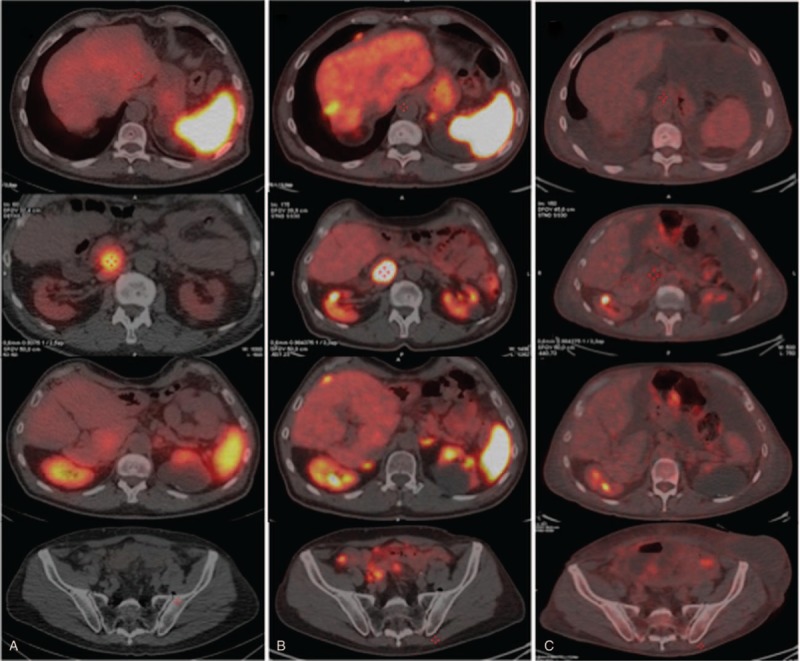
Restaging with ^111^In-Octreotide SPECT/CT scan (column a), ^68^Gallium-DOTATOC (column b), and ^18^F-FDG PET/CT scan (column c) of the patient, performed after colectomy and partial right hepatectomy and subsequent treatment by “cold” somatostatin. Since NET is a G2 tumor (Ki67 index 12%), positivity can observed in columns a and b. ^111^In-Octreotide SPECT-CT scan (column a) showed persistence of the retropancreatic uptake without other significant uptake areas. ^68^Ga-DOTATOC PET/CT scan (column b) confirmed uptake behind the pancreas head (SUV max 56.8) and showed multiple nodules of peritoneal implants in the periglissonian space, omentum, mesenteric region and pelvis (SUV max 13.8-15.4). ^18^F-FDG PET/CT scan (column c): metabolic activity in retropancreatic lesion was absent (SUV max 1.9) and mild pathological nodal uptake was observed in mesenteric region, with a conspicuous ascitic and pleural effusions.
